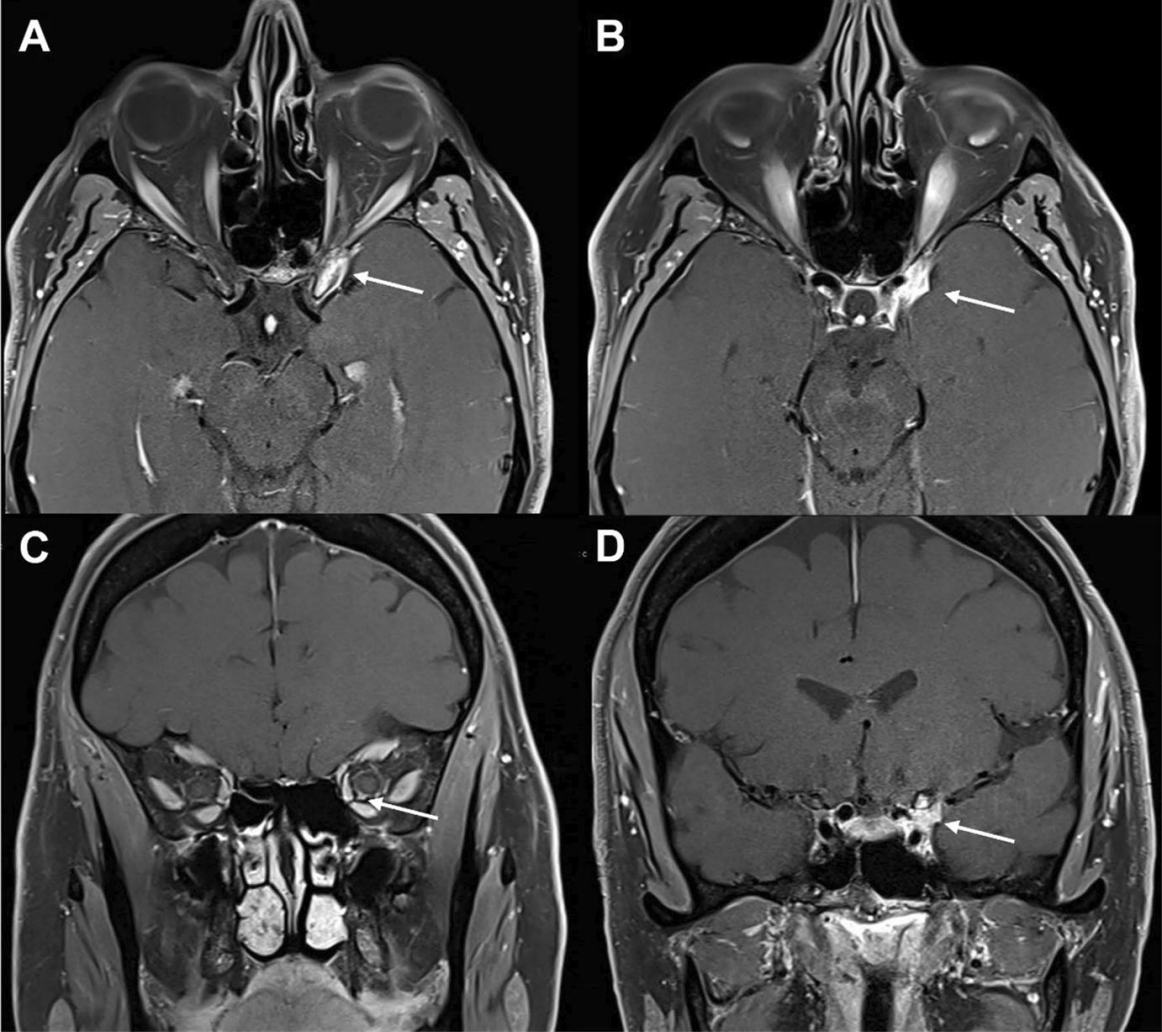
T1-weighted, fat-suppressed post-contrast MRI scans for Case 1, demonstrating the enhancing infiltration of the left cavernous sinus with extension through the superior orbital fissure to orbital apex with apical nerve sheath enhancement (white arrows)
